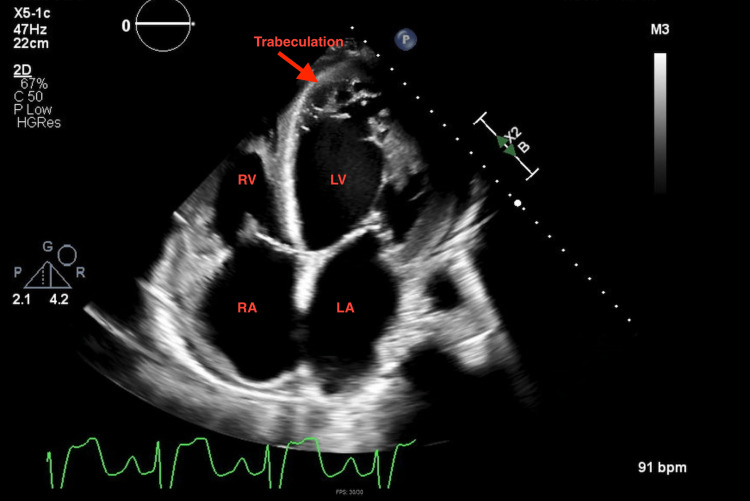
2D transthoracic echocardiogram (four-chamber view) showing a region of trabeculation at the left ventricular apex
